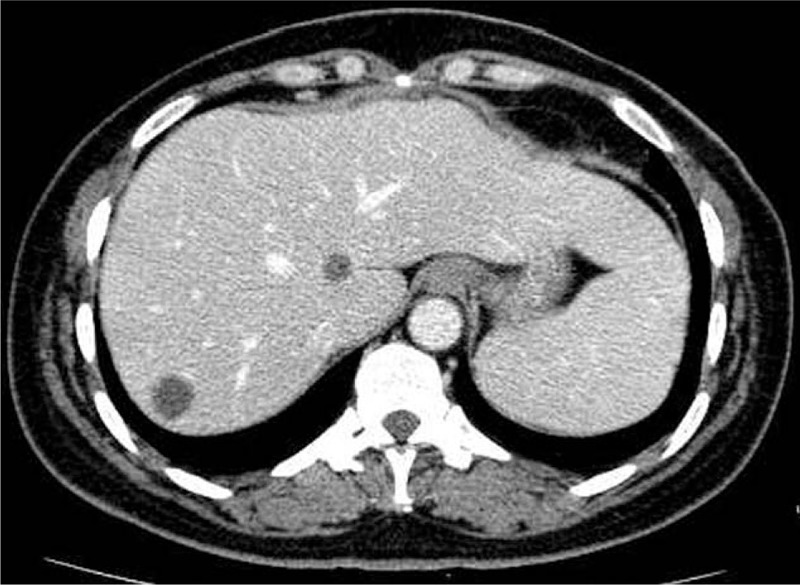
Abdominal computed tomography scan showing the recurrent small cystic lesions were extremely similar to simple cysts.
